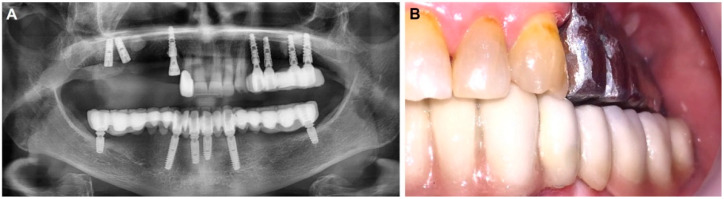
Provisional prosthesis was mounted in the same session after sinus lifting using Paste-CPC. The prosthetic stage started on the tenth week. (A) Orthopantomagram shows the metal prosthesis on the right side, and the cement is seen near the cranial end of the implants. (B) The metal framework try-in on the right side.
